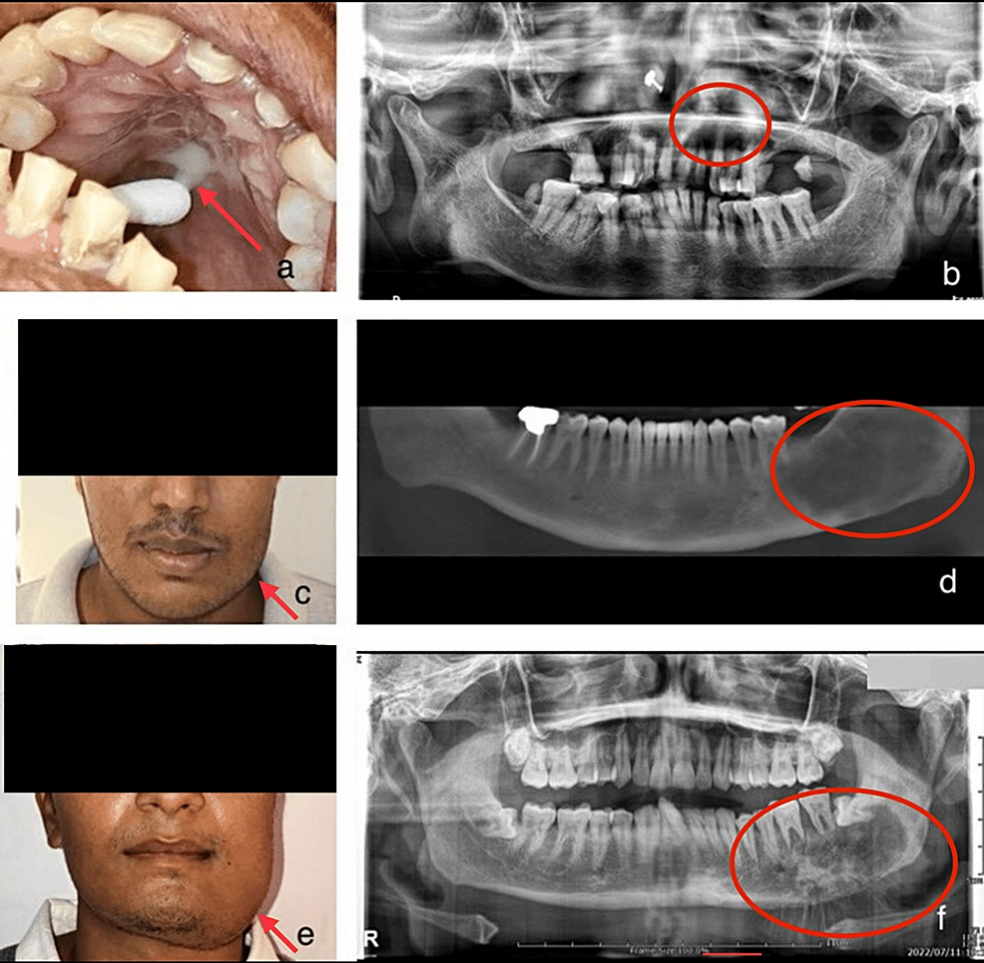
Figures showing clinical and radiographic images a) Purulent white discharge in the palatal region of fungal osteomyelitis - mucormycosis b) Orthopantomogram revealing a hazy radiolucency extending from 21 to 25 region in the maxilla of fungal osteomyelitis - mucormycosis c) Swelling involving the left lower side of the face - ossifying fibroma d) Cone beam computed tomography (CBCT) revealing a mixed lesion with indistinct borders extending from the distal part of 36 and extending posteriorly to the ramus of the mandible - ossifying fibroma e) Facial asymmetry involving the left lower part of the face - osteosarcoma f) Orthopantomogram revealing a typical sunburst appearance of a mixed osteolytic lesion on the left posterior mandible with indistinct borders - osteosarcoma
